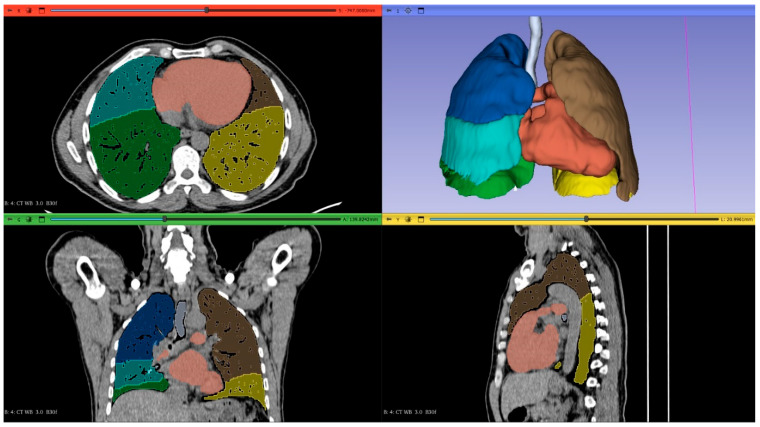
Preoperative 3D−CT volume measurement and visualization of both lungs in a patient scheduled for pneumonectomy.
